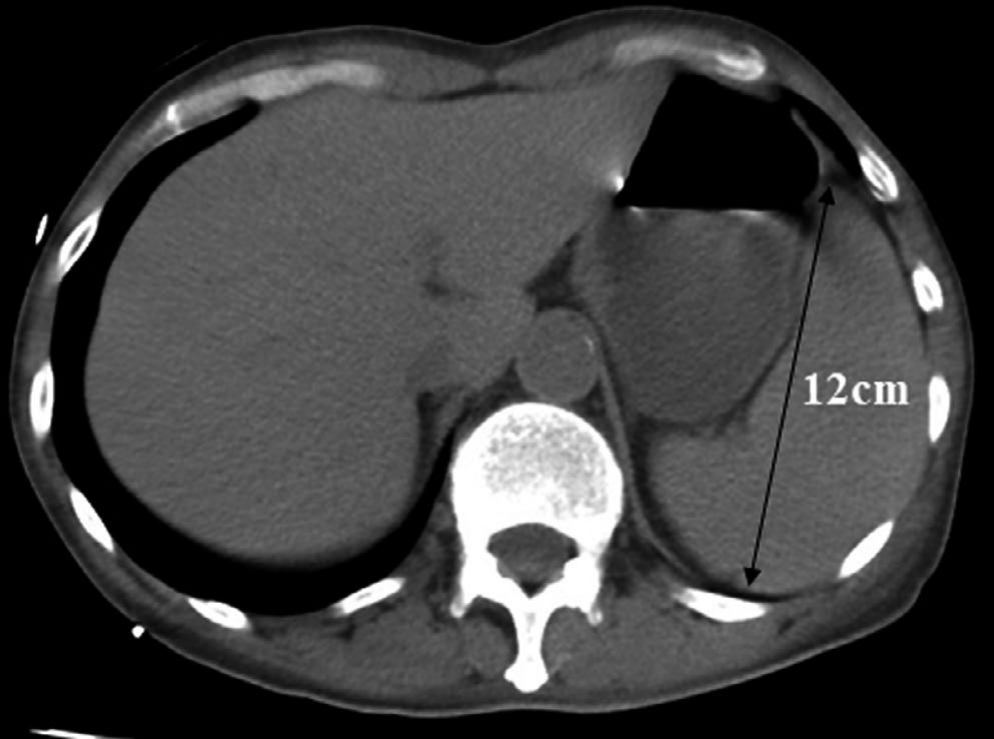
Computed tomography scan showing splenomegaly
